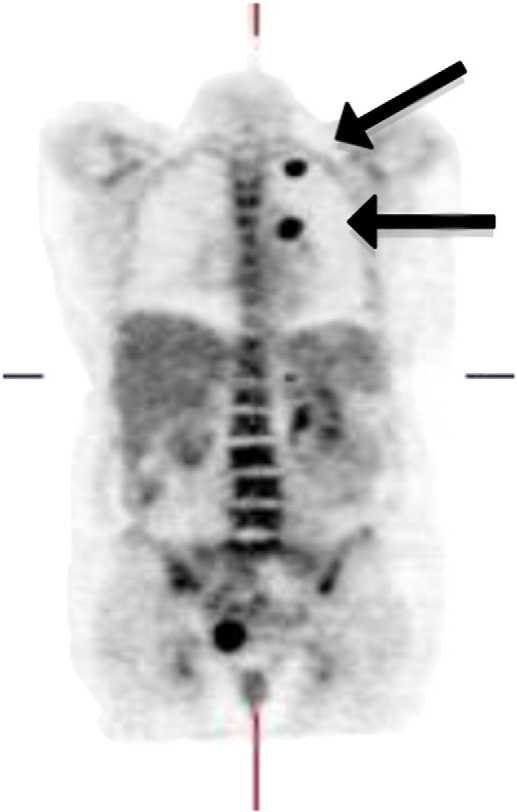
Fused PET/CT sagittal section showing two hypermetabolic lung nodules (marked with arrows).
